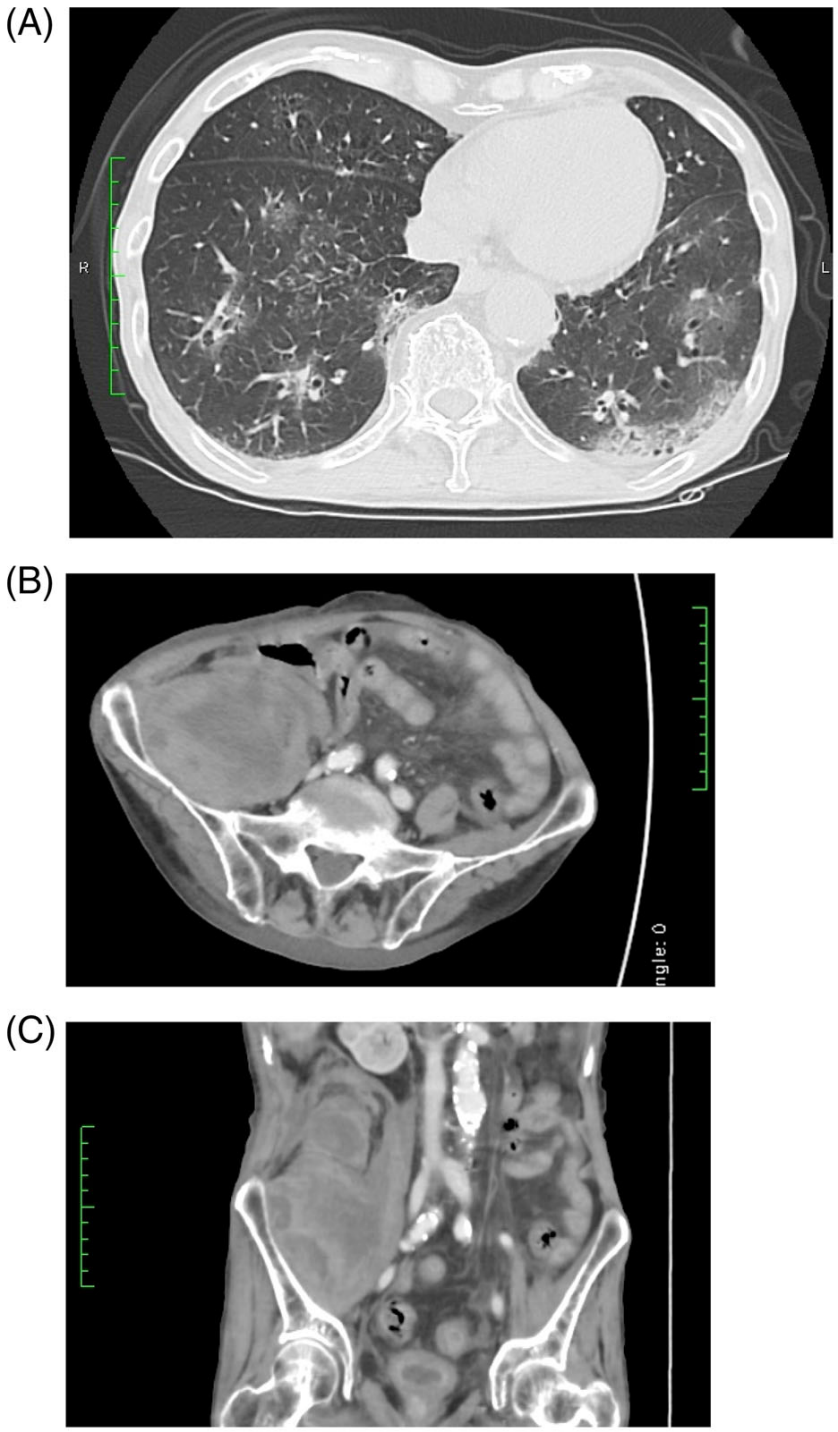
(A) Chest high‐resolution computed tomography revealing bilateral and multifocal ground‐glass opacities in predominately peripheral distribution. (B and C) Contrast‐enhanced abdominal and pelvic computed tomography showing a massive haematoma (6.0 × 9.5 × 10.5 cm) in the right iliopsoas muscle spreading to the retroperitoneal space
